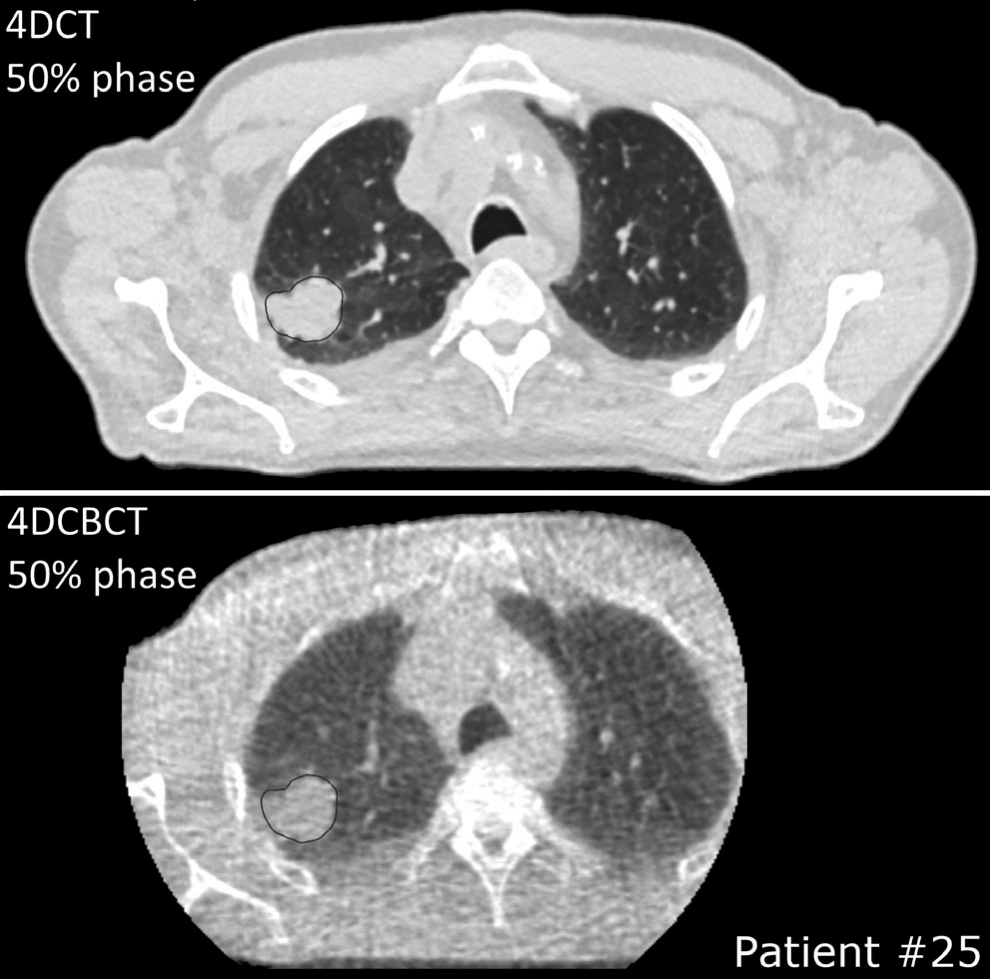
Example of a delineated GTV (transverse view) after deformable warping and correction on a 4DCT phase and the corresponding phase on a 4DCBCT scan.
